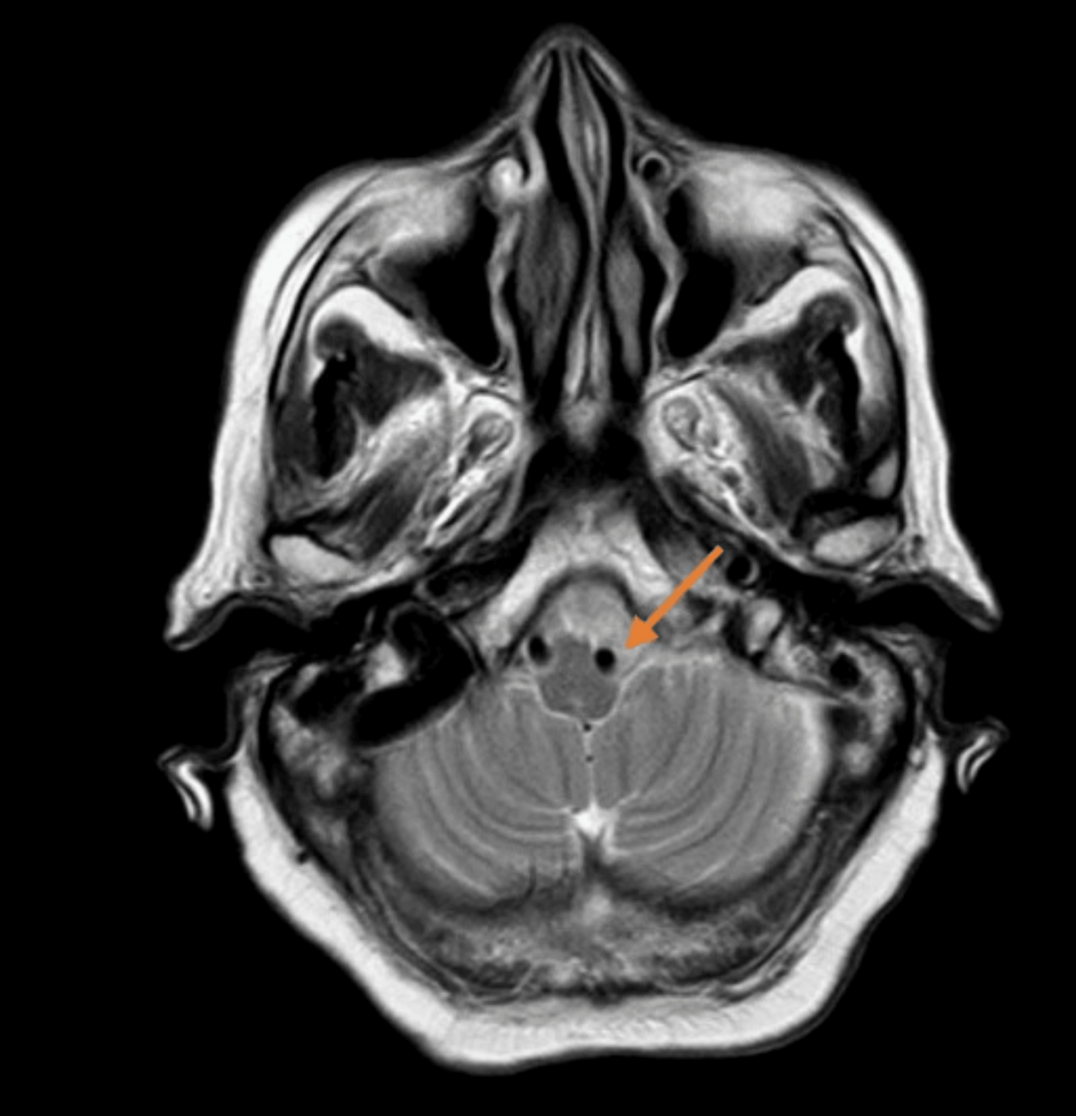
T2 weighted image of the brain-axial section showing ectatic left vertebral artery compressing the left anterolateral medulla T2WI, T2-weighted image
